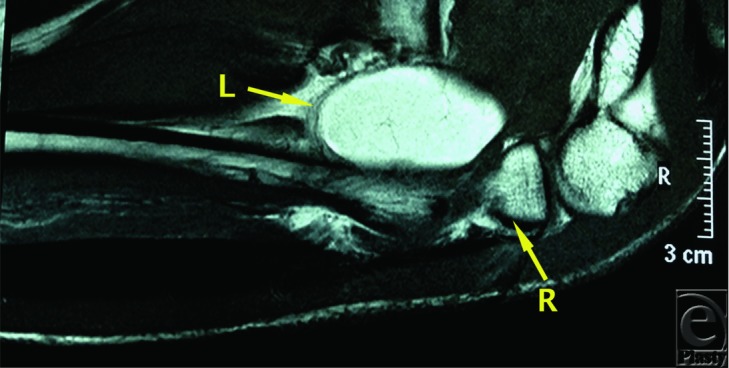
T2 turbo spin-echo weighted magnetic resonance imaging demonstrating a space-occupying lesion in the radial tunnel. L indicates lesion; R, proximal radius.
